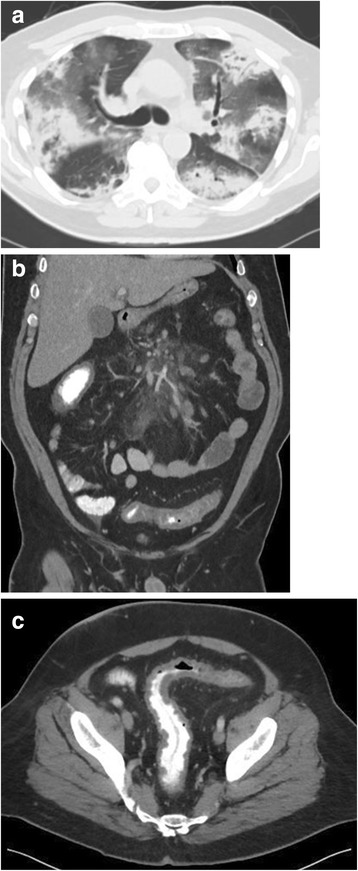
a 78-year-old female patient with CLL on treatment with Idelalisib with pneumonitis, axial CT of the chest obtained in arterial phase demonstrates bilateral patchy areas of ground glass and consolidative opacities. b and c 55-year-old male patient on Idelalisib therapy with colitis, reconstructed coronal CT images of the abdomen and pelvis in portal venous phase demonstrate short segment circumferential thickening of the colonic loop with mildly enlarged mesenteric lymph nodes and panniculitis. Axial CT images of the pelvis in portal venous phase show circumferential wall thickening of the sigmoid colon
